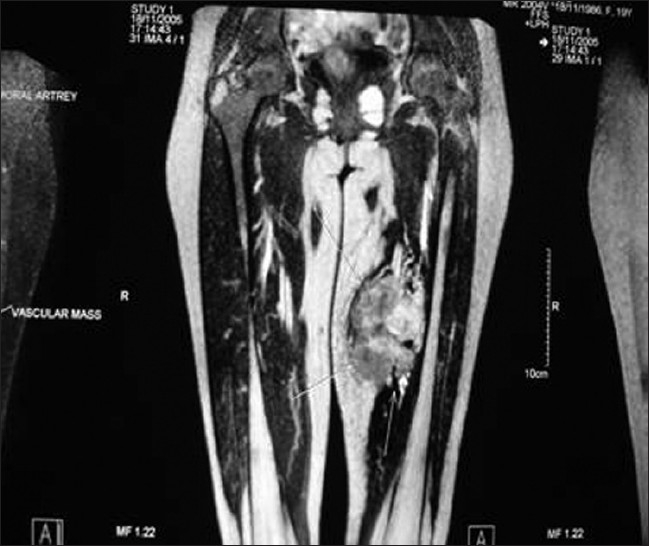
Coronal T2W MRI of patient 1 showing mass involving vessel
